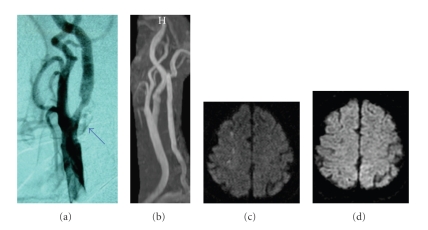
(a) Peroperative common carotid angiogram (lateral projection) of a 64-year-old asymptomatic patient with a 60% stenosis of the right ICA by NASCET criteria (*arrow*). (b) Postoperative MR angiography confirmed disappearance of the stenosis. (c) Axial diffusion-weighted MR imaging of the brain immediately after the occurrence of neurologic events following the DSA. Ipsilateral hyperintense lesions are appreciable at the cortical-subcortical junction of right front-parietal lobes. (d) Postoperative diffusion-weighted imaging indicative of no ischemic lesions associated with CEA. Postoperative MR imagings ((b) and (d)) were performed on the next day after CEA.
